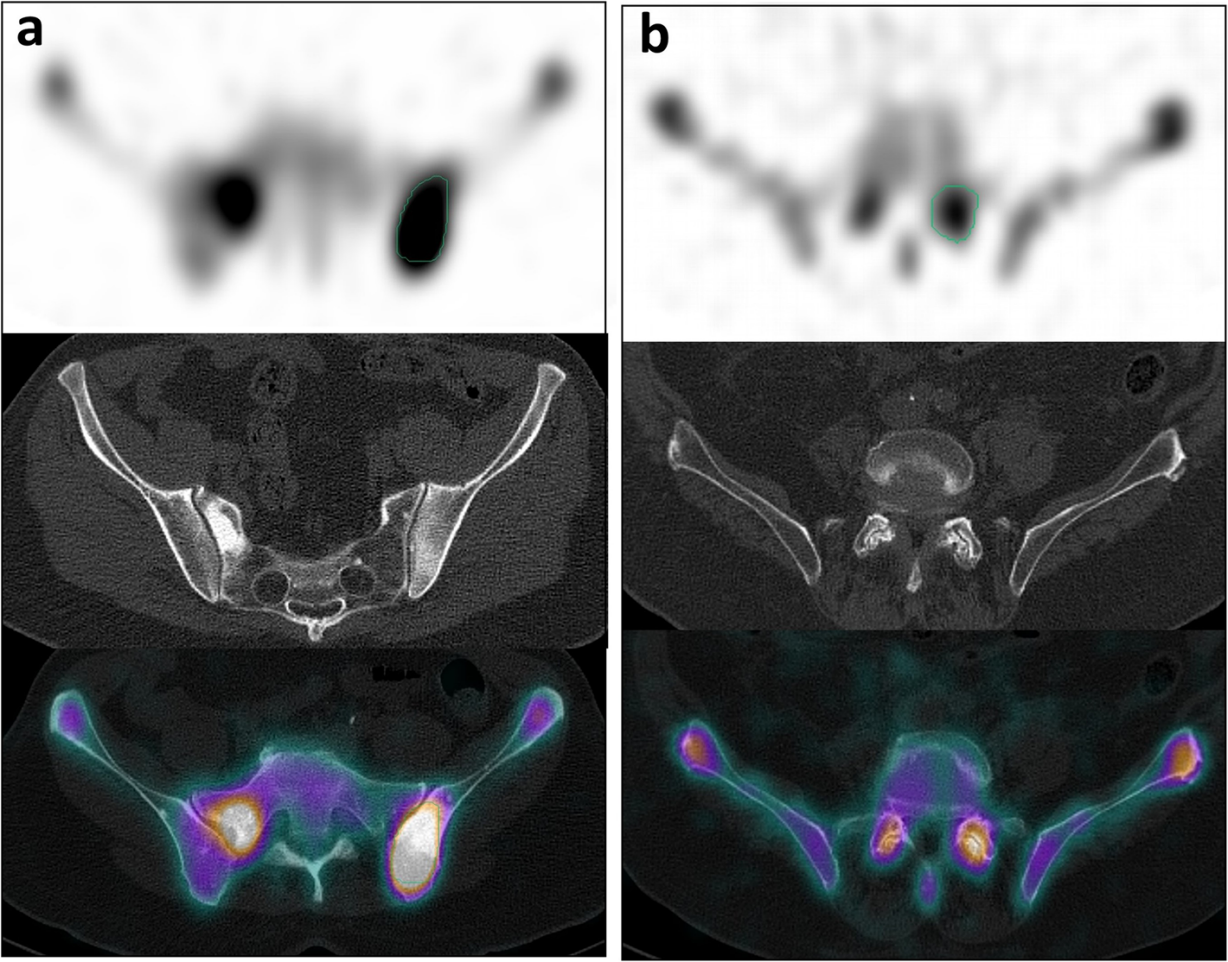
a Osteoblastic lesion of the pelvis in a 57-year-old male patient known for prostate cancer, showing a high SUV_max_ of 28 g/mL and SUV_mean_ of 17 g/mL. b Lumbar spine osteoarthritic changes of the L4-L5 facet joints in a 83-year-old male patient with hip pain, showing SUV_max_ of 15 and 13 g/mL and SUV_mean_ of 9.0 and 7.8 g/mL in the left and right facet joints, respectively
